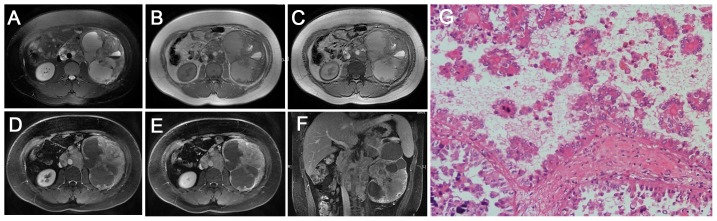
Translocation RCC in a 13-year-old boy (patient 6 in Tables 1–2) with internal gross hematuria and a left cervical mass (biopsy proved metastases). A–C, Axial T2WI and plain (non-contrast) T1WI (B, IP; C, OP) showing a large, ill-defined, irregular mass (T2, high-low mixed signal intensity; T1, iso-high mixed signal intensity; liquid-gas surface) with a large, patchy hemorrhage and necrosis in the center of the left renal mass, without an integrated capsule. Multiple retroperitoneal metastases are detected. D–E, CMP and NP gradient-echo MR images showing marked enhancement, predominantly in the periphery of the mass. F, Coronal delay phase image showing marked enhancement in the periphery of the mass, invading the left renal pelvic space and vessels. G, The tumor cells contain hyaline cytoplasm and show aciniform, papillary and micro-papillary structures (HE 10 & 20).
